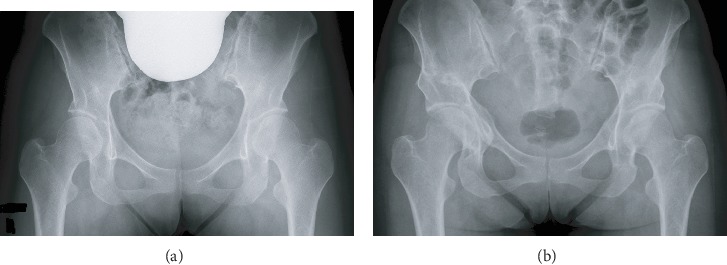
Radiographs. (a) Preoperative pelvic radiograph showing bilateral acetabular dysplasia. (b) Pelvic radiograph after bilateral curved periacetabular osteotomy. At 12 months postoperatively, complete union was observed in both sides.
